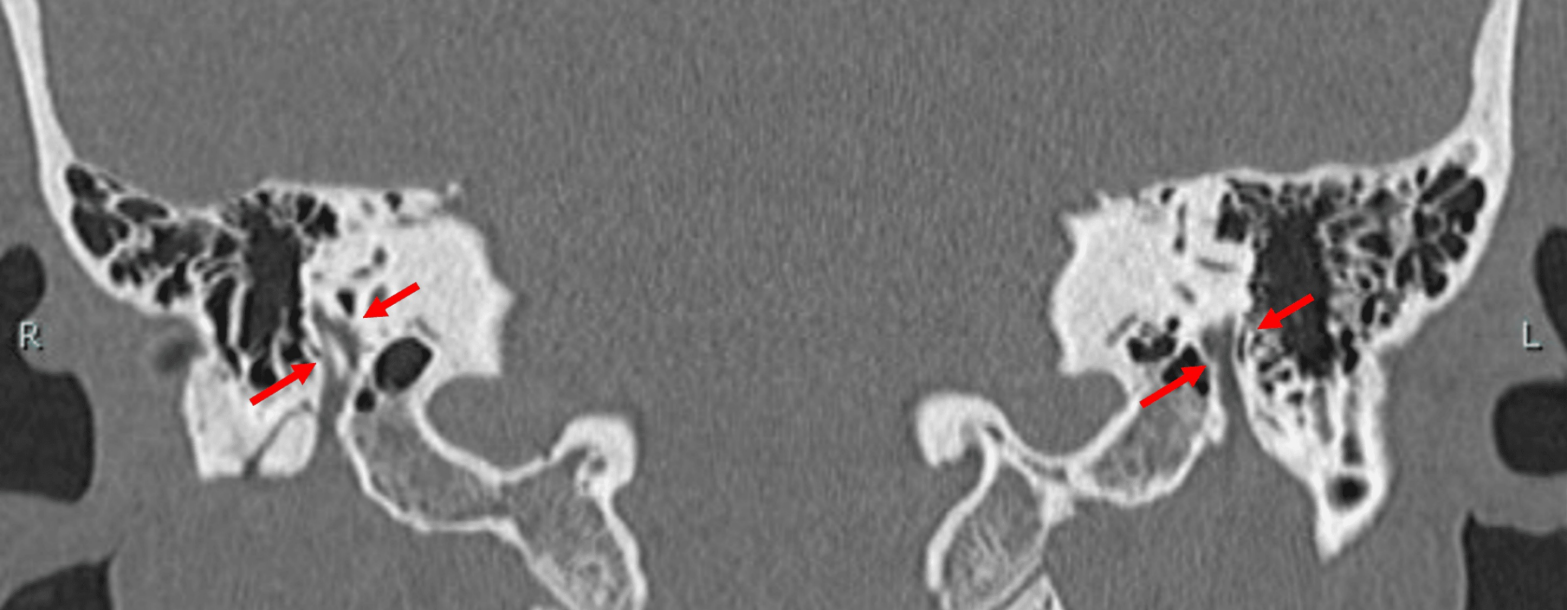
Coronal HRCT image of the temporal bone showing the mastoid segment of facial nerve appearing as branched fiber (arrows) on both (R = right and L = left) ears (Case 2). HRCT, High-resolution computed tomography
